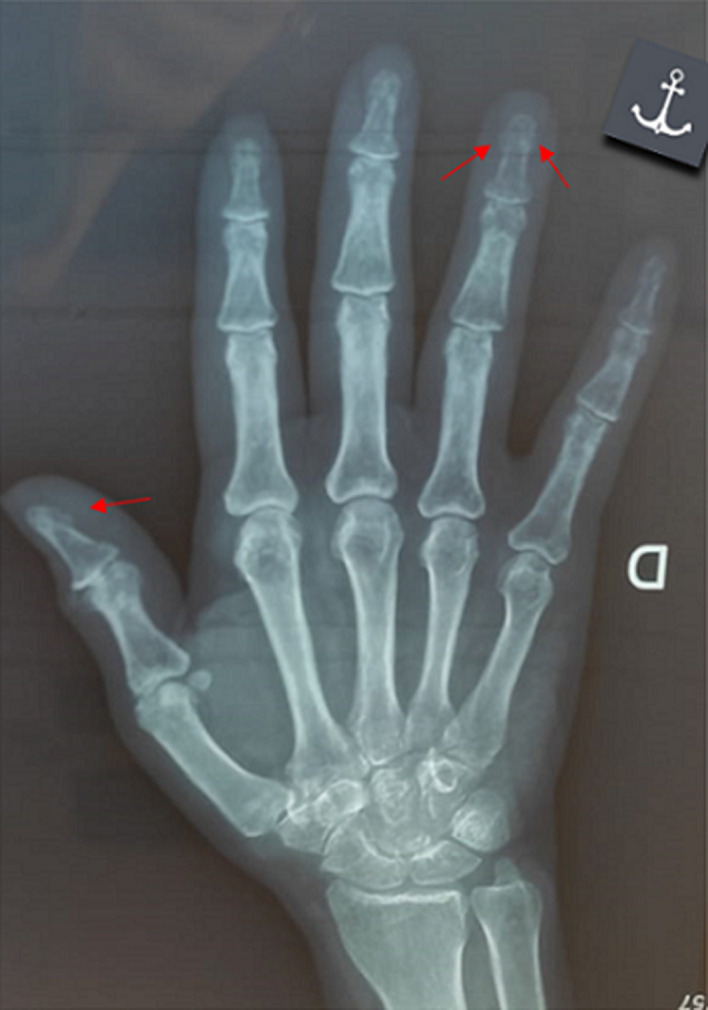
X-ray of the hand showing a sea anchor osteophytosis
